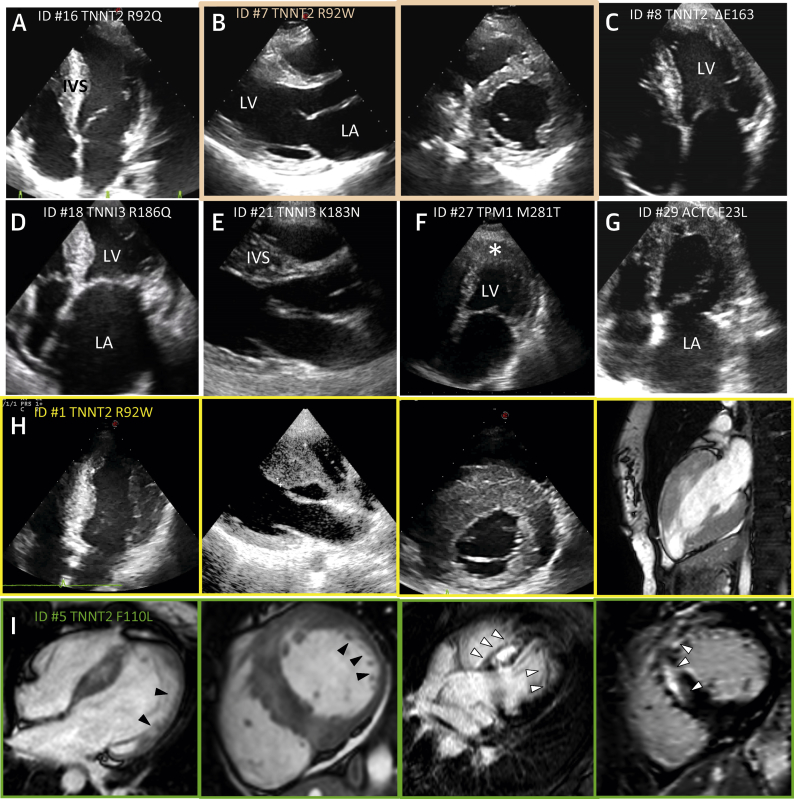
Phenotypic Variability in Thin-Filament–Associated HCM Echocardiographic and cardiomagnetic resonance (CMR) images showing variable extent and distribution of left ventricular hypertrophy (LVH) in patients from the thin-filament study cohort (patient’s ID number and mutation are indicated). (A) Moderate LVH associated with TNNT2-R92Q, with classic localization at the interventricular septum (IVS). (B) Mild midseptal hypertrophy and marked left atrial (LA) dilation associated with TNNT2-ΔE163. (C) Mild concentric LVH associated with TNNT2-R92W. (D) Moderate midseptal LVH with small LV cavity size and severe LA dilation (restrictive evolution) associated with TNNI3-R186Q. (E) Classic asymmetric septal LVH associated with TNNI3-K183M. (F) Apical LVH associated with TPM-M281T; ∗indicates LV apex. (G) Apical LVH with severe LA dilation associated with ACTC-F23L. (H) Markedly asymmetric LVH involving the anterior and posterior septum and anterior wall associated with TNNT2-R92W. (I) CMR images from a patient carrying the TNNT2-F110L mutation. (From left) Four-chamber and midventricular short-axis views showing extensive regions of noncompaction (black arrowheads) in the LV apical and free wall regions; 4-chamber and short-axis views showing large areas of late gadolinium enhancement (white arrowheads) in the anterior free wall and in the septum, respectively. HCM = hypertrophic cardiomyopathy.
